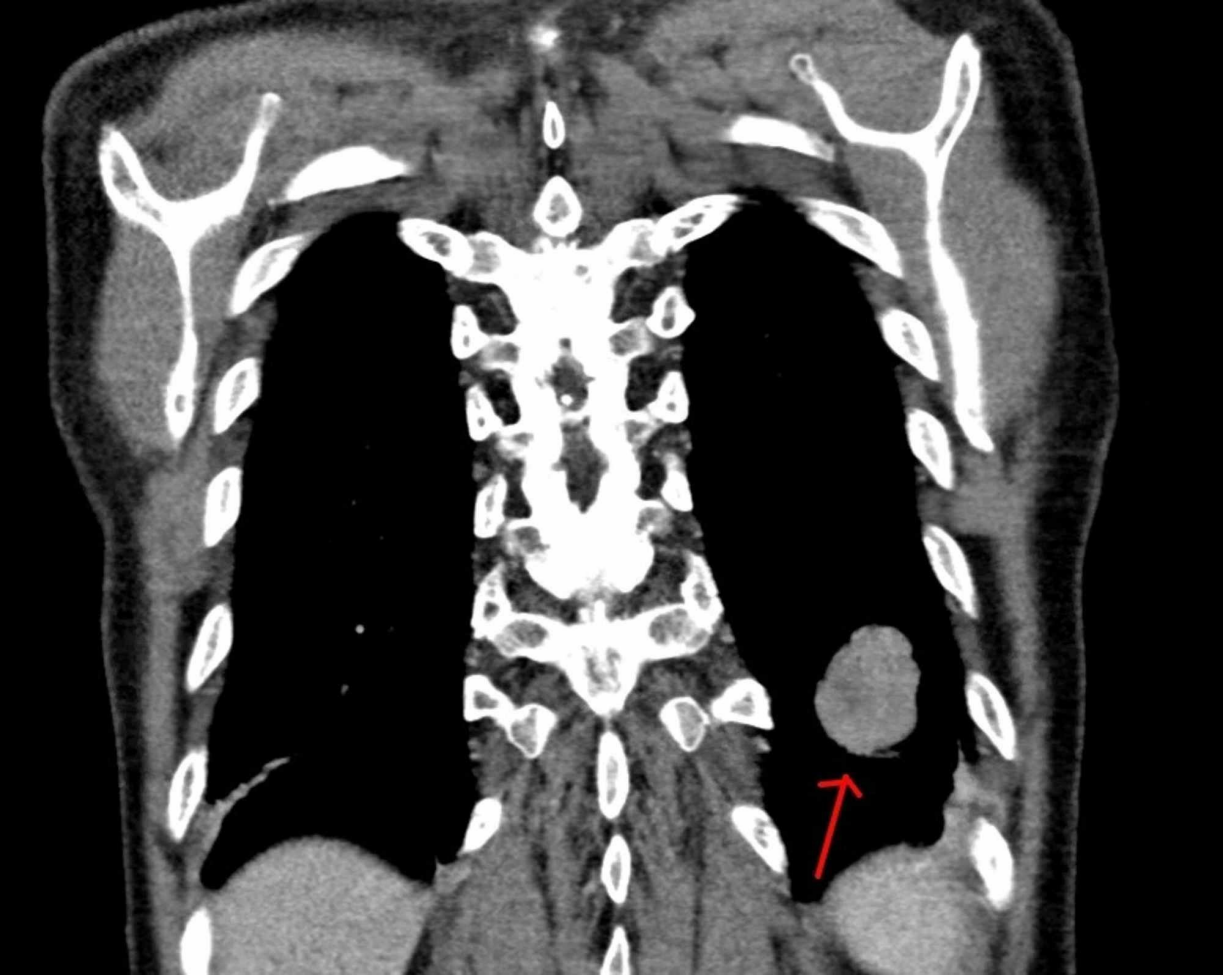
CT chest abdomen and pelvis
